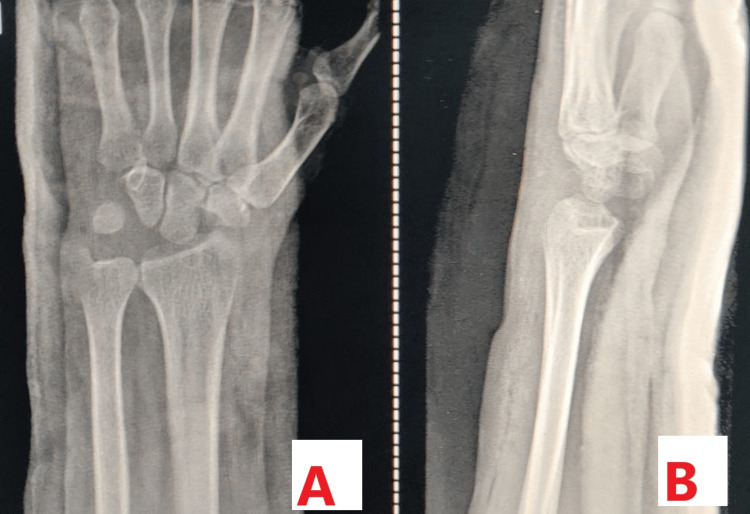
Postoperative radiograph of the wrist joint. A: Posteroanterior view of the wrist joint. B: Lateral view of the wrist joint.
